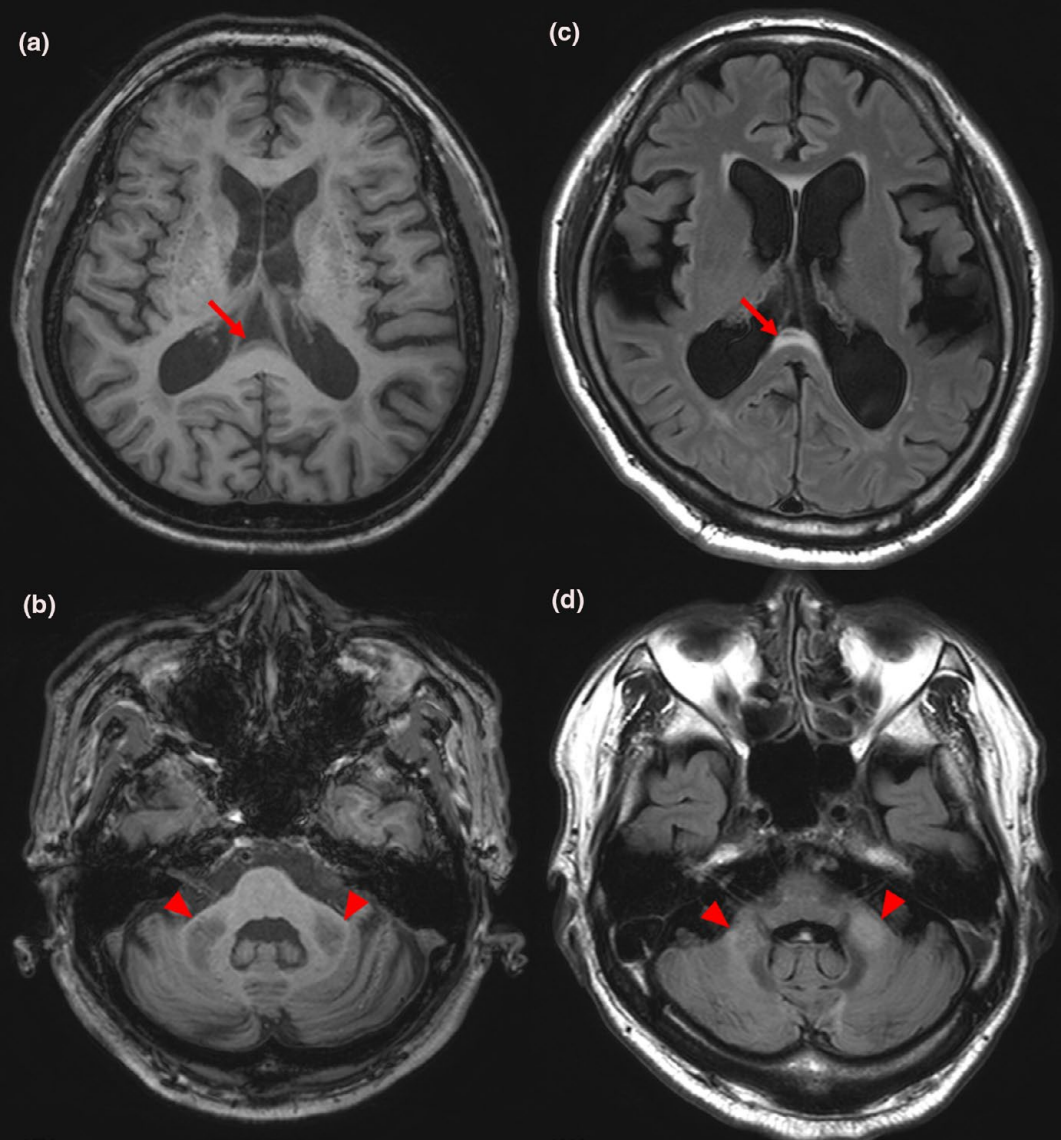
Magnetic resonance images of patients with *FMR1* premutation. Panels (a) and (b) are images of patient 1, and panels (c) and (d) are images of patient 2. Panels (a) and (c) shows corpus callosum splenium sign (red arrows), and panels (b) and (d) shows middle cerebellar peduncle sign (red arrowheads)
